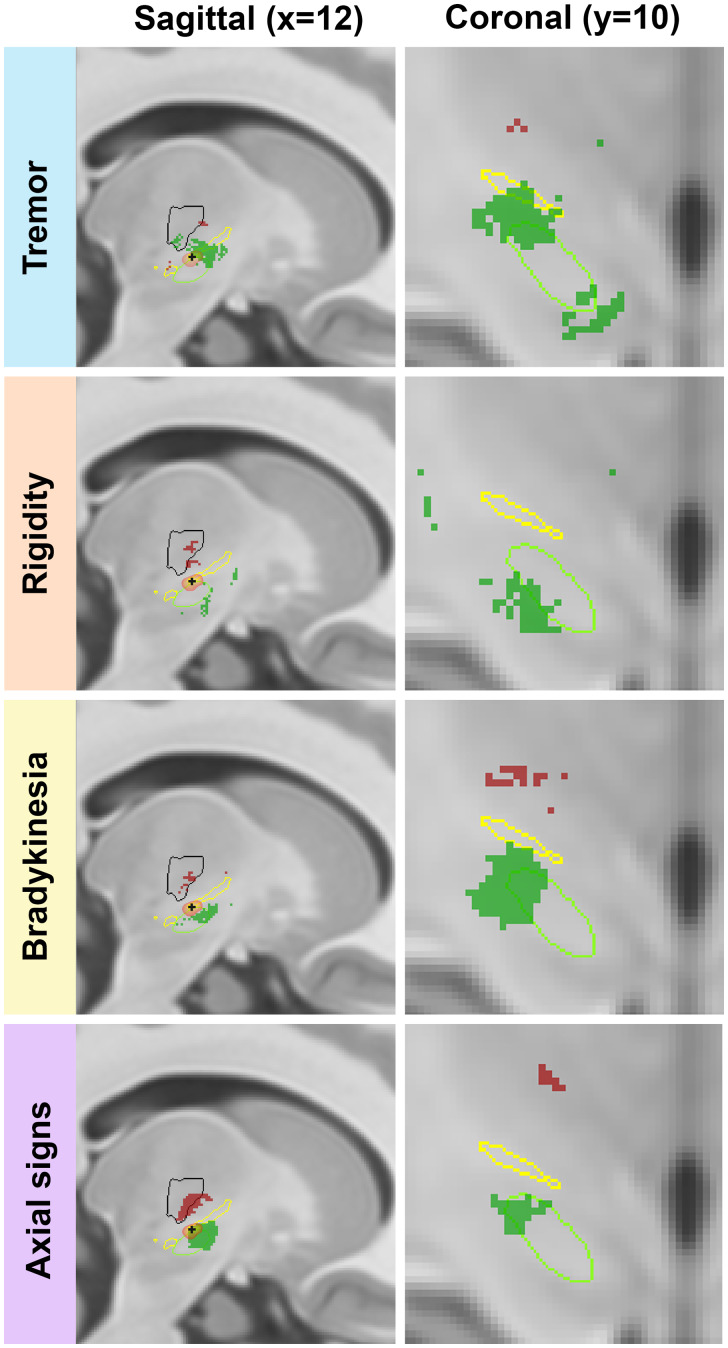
Sign-specific area of clinical change after STN-DBS. Binarized areas of optimal (‘hot spots’) and suboptimal (‘cold spots’) efficacy were identified using mass univariate analysis (uncorrected *P* < 0.01). Hot (positive *t*-values, green) and cold (negative *t*-values, red) spots are shown. The subthalamic nucleus (green outline), zona incerta (yellow outline), and thalamic ventral-intermediate nucleus (black outline) are projected on sagittal (first column) and coronal (second column) T_1_-weighted MRI (MNI ICBM 2009 b NLIN asymmetric). The nuclei outline were derived from nuclei labels^18^ using FSLeyes for visualization.
